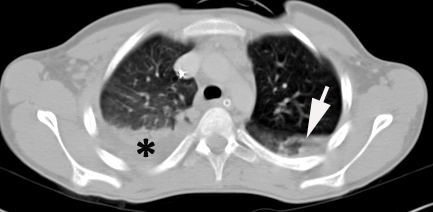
Thoracic CT. Arrow – lung contusion. Hemothorax
